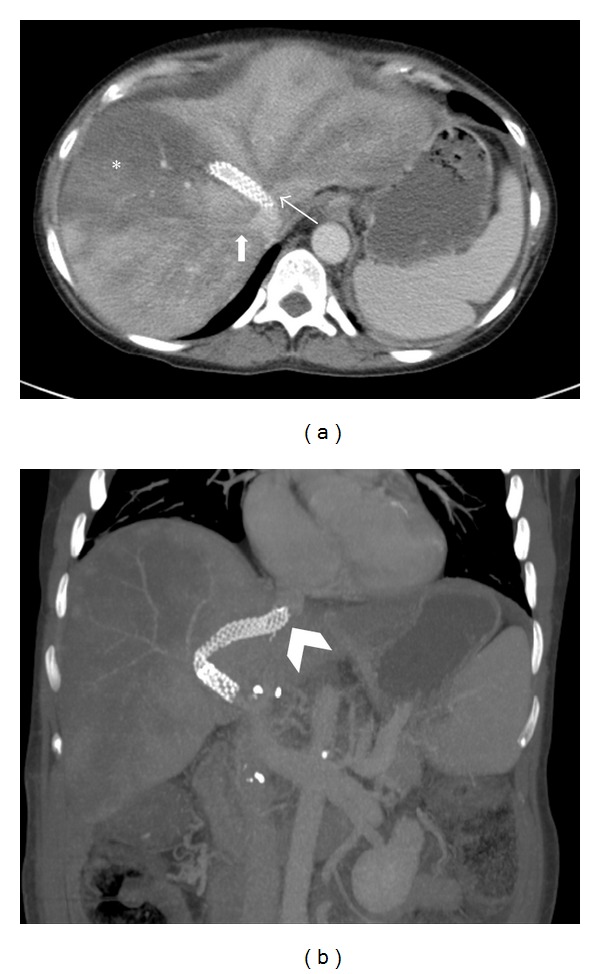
(a) Axial contrast-enhanced CT of the upper abdomen during the portal-venous phase demonstrates thrombosed left and middle hepatic veins (*thin arrows*). The proximal end of the TIPS stent is seen within the IVC which is occluding ostia of the left and middle hepatic veins (*black arrow*). The right hepatic vein is patent and adequately opacified with IV contrast (*thick arrow*). A wedge-shaped hypodense area in hepatic segments V and VIII (*asterisk*) representing hepatic infarction. (b) Coronal MIP image of the upper abdomen shows the position of the TIPS stent, communicating the right portal vein and the mid hepatic vein with its most cranial tip within the IVC (*arrowhead*).
